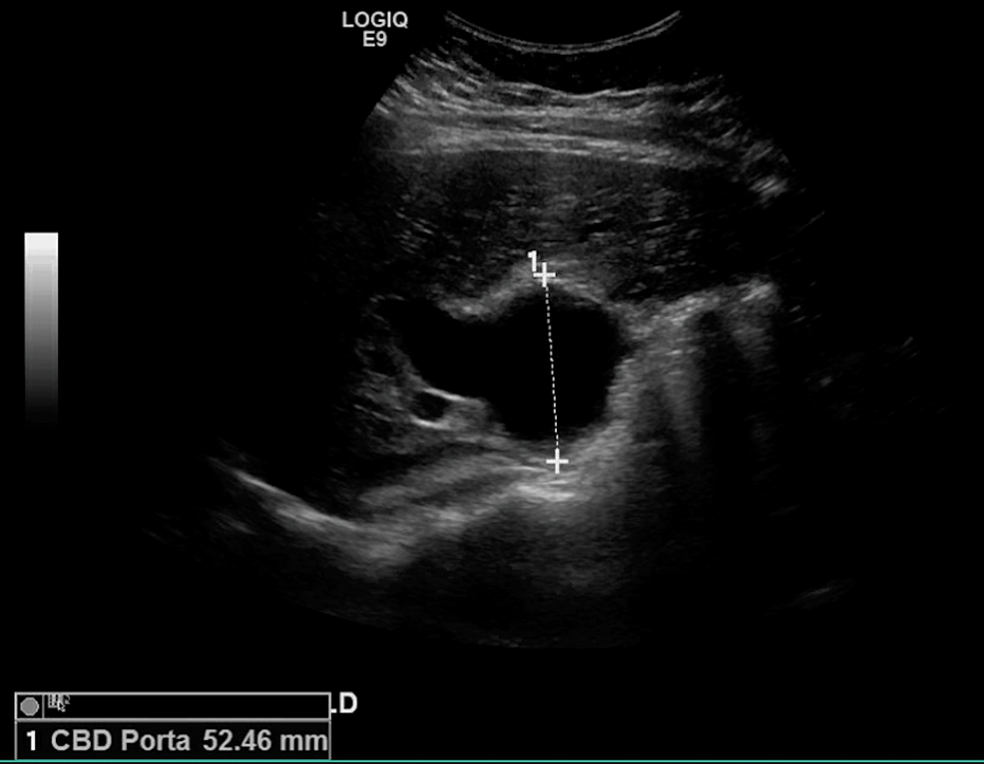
RUQ US showing significantly dilated CBD RUQ: right upper quadrant; US: ultrasound; CBD: Common bile duct
